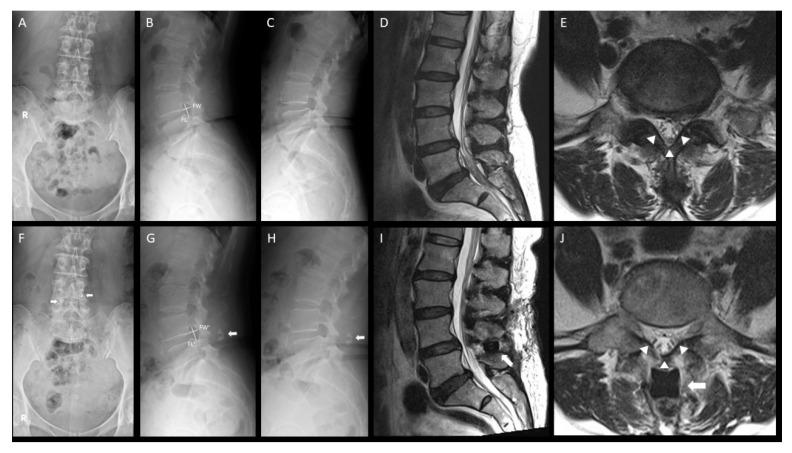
Radiographs and MRI of a 60 year-old-woman with spinal stenosis at L4–5. (A–C) Preoperative radiographs showed deceased disc height at L4–5 with mild spondylolisthesis. Measurement of Foraminal Length (FL) and Foraminal Width (FW) were demonstrated. (D,E) MRI showed L4–5 spinal stenosis and dark signal in the disk anterior to left lateral recess. (F–H) After decompression surgery and DIAM implantation, postoperative radiographs showed increased disc height and preserved segmental mobility. (I,J) Postoperative MRI showed increased disc height and enlarged neuroforamen (arrowheads). DIAM implants were well fixed postoperatively (arrows in (F–J)). Abbreviations: FL’ = postoperative foraminal length; FW’ = postoperative foraminal width.
